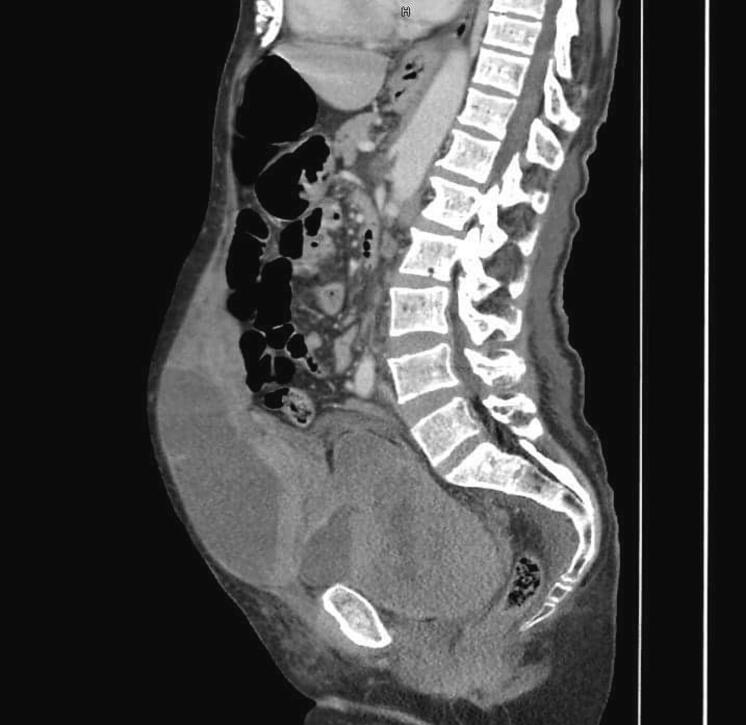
Sagittal section of a CT scan showing the second hematoma in the left psoas muscle.
